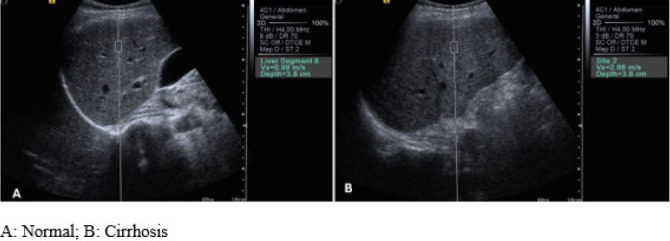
Example of ultrasound-based liver elastography using the pSWE technique.
